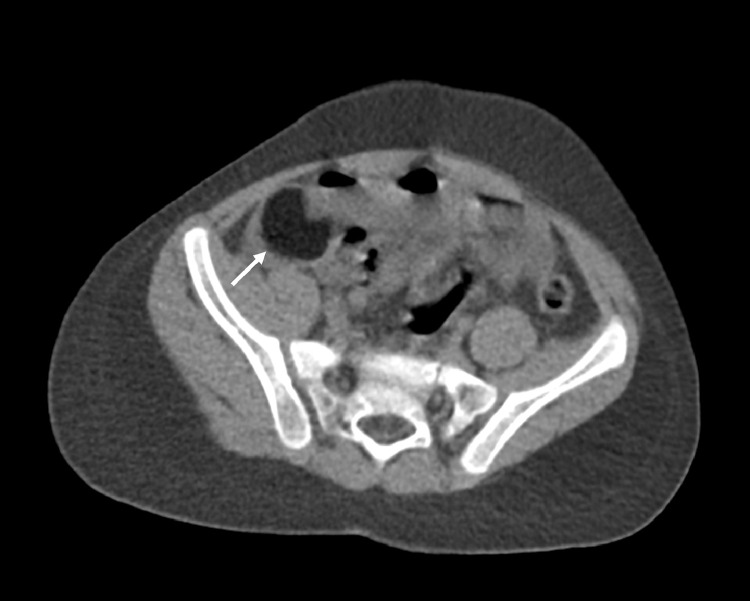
Subsequently, computed tomography (CT) of the abdomen was performed. Axial image demonstrates a 1.8 × 2.9 × 4.3 cm well-circumscribed hypodense mass in the terminal ileum (white arrow), resulting in upstream small bowel obstruction.
